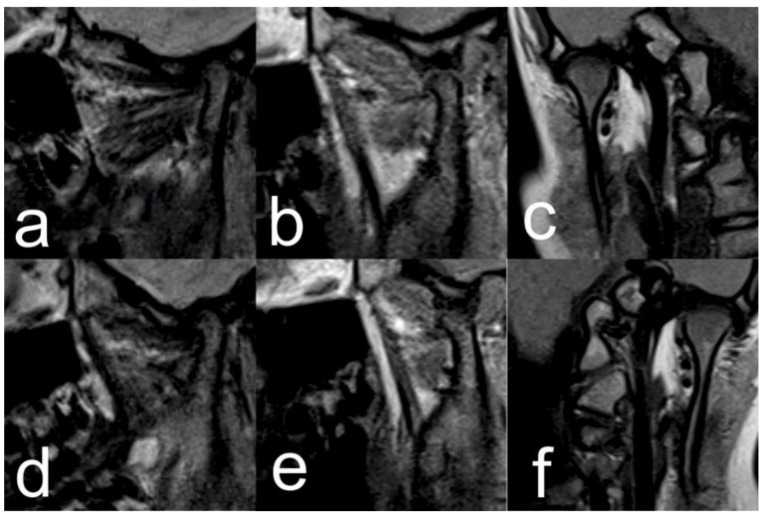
Pre-treatment TMJ MRI: Right TMJ sagittal view closed mouth (a), open mouth (b) and closed mouth coronal view (c); Left TMJ sagittal view closed mouth (d), open mouth (e), closed mouth coronal view (f).
